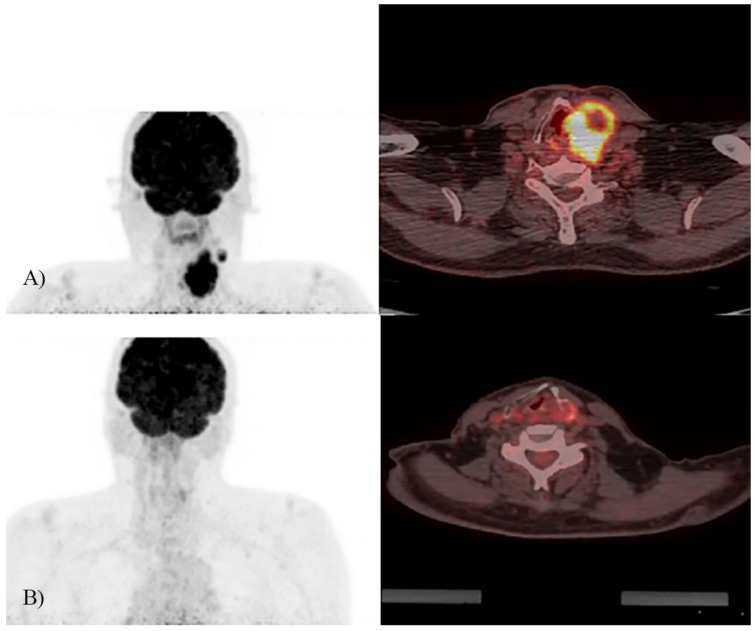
A representative LA-SCCHN case of good response (>50% visual tumor volume reduction) to induction chemotherapy. (A) Pre-ICT 18F-FDG PET/CT; (B) post-ICT 18F-FDG PET/CT of the same patients with cT4N1M0 squamous cell carcinoma of the left pyriform sinus.
